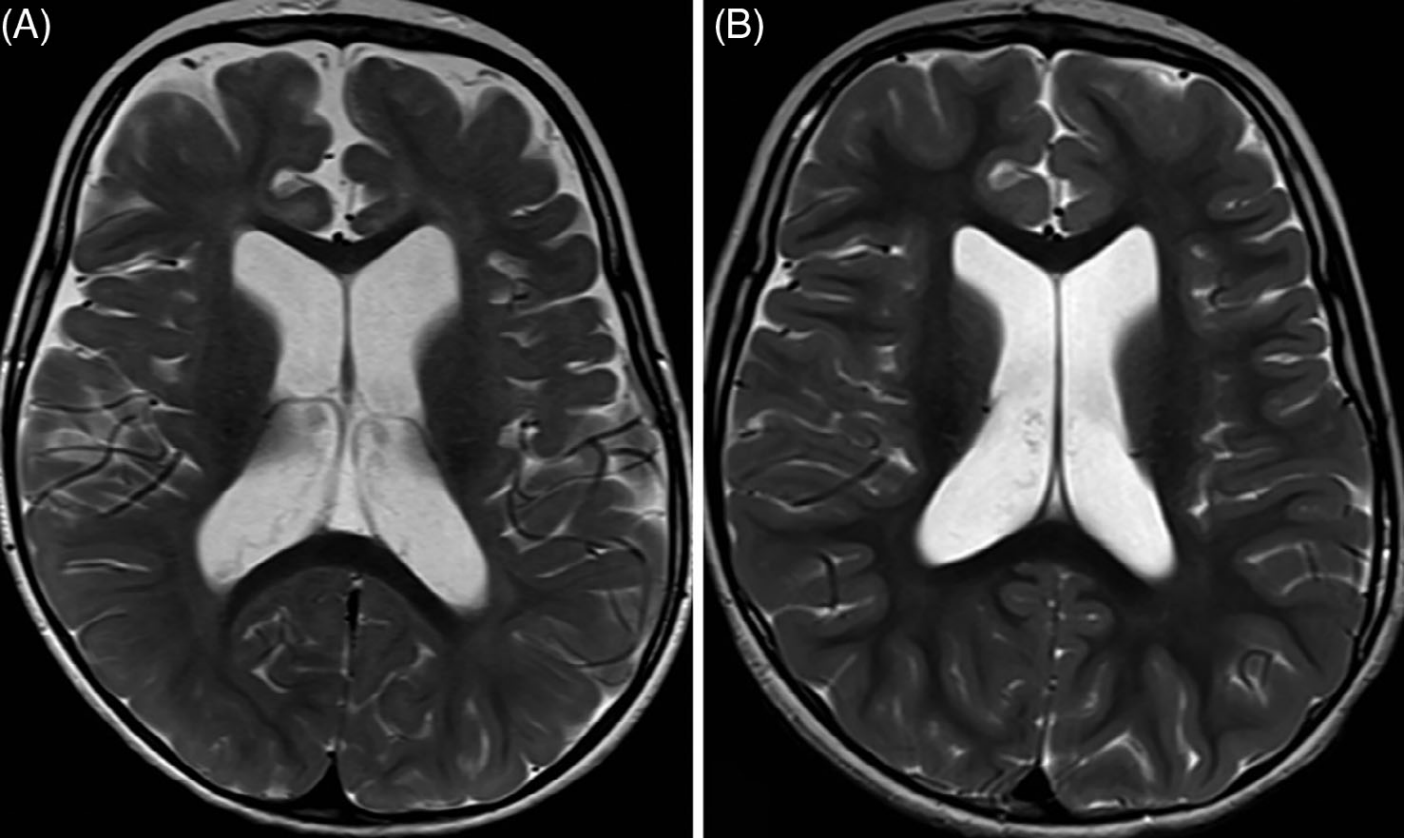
Brain MRI. T2‐weighted images at age of 24 months (A) and 5 years (B) show lateral ventricles and cerebral sulci that are mildly prominent for age
